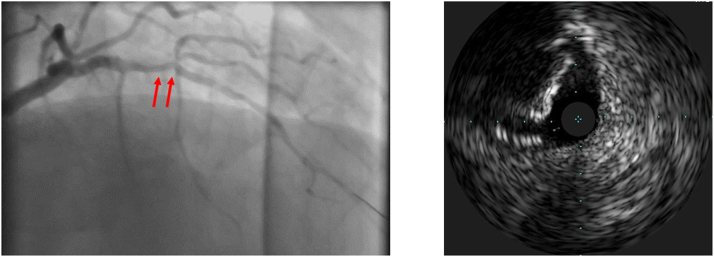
Coronary Angiography and Intravascular Ultrasound (Left) Coronary angiography RAO cranial view shows a significant calcified lesion in the mid-LAD (arrows), and (Right) intravascular ultrasound of the mid-LAD shows a calcified nodule. LAD = left anterior descending artery; RAO = right anterior oblique.
